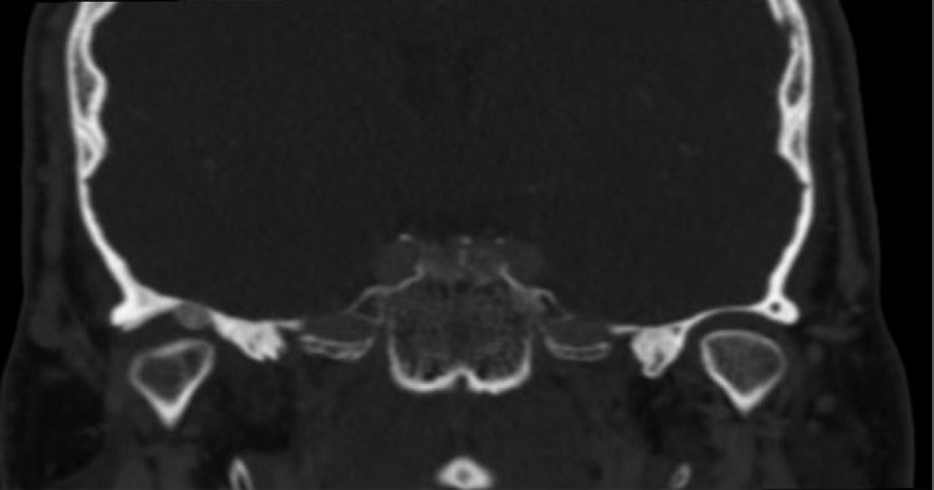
Pigmented villonodular sinovitis of TMJ. CT image (right size).
